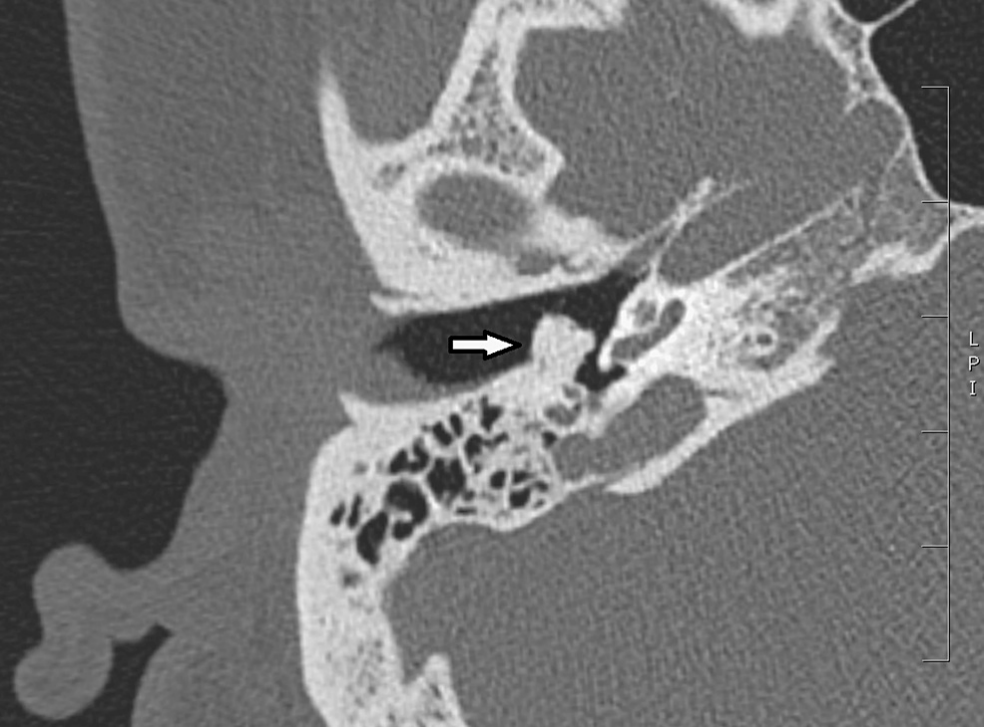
Axial view of right ear on CT imaging, with an arrow highlighting opacification arising from retrotympanum, lateral to aditus.
